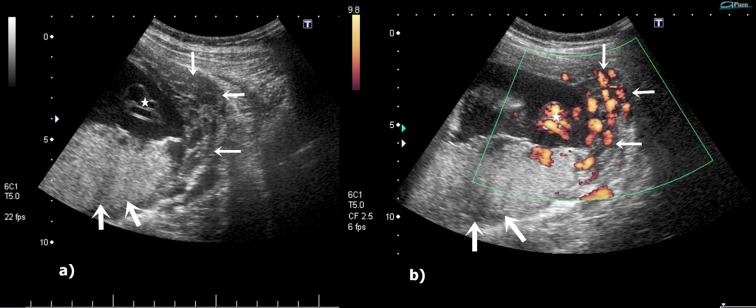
Gray scale and power Doppler US demonstrates varicosities extending into the myometrium (white arrows). Placenta is shown with thick arrows and umbilical vessels with star respectively (a, b).
